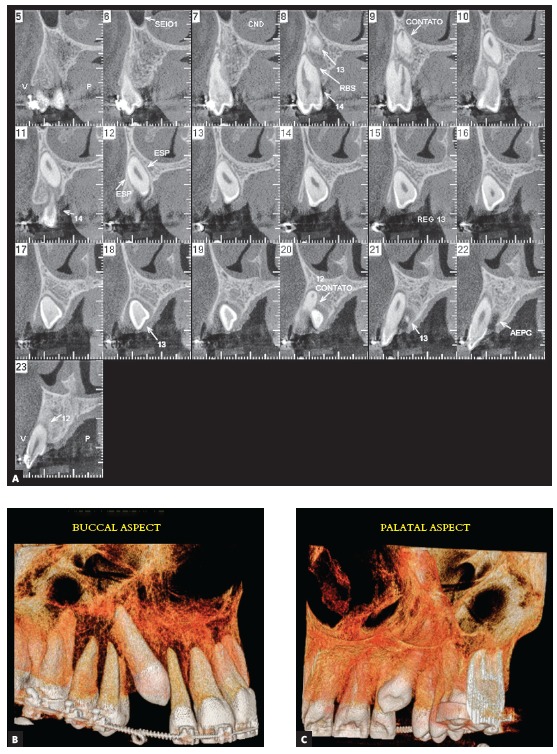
CT scan: (A) oblique cross-sectional slices and 3D reconstructions, buccal aspec (B) and palatal aspect (C).
